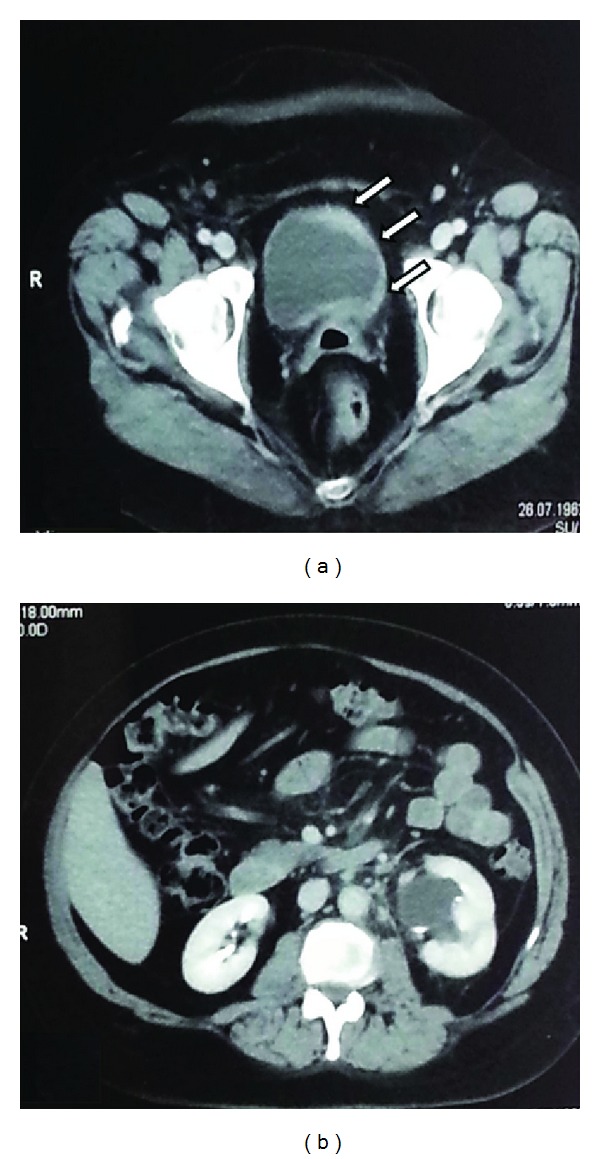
Abdominal computed tomography scanning showing (a) thickening of the left bladder wall (arrows) with grade II left hydronephrosis (b).
